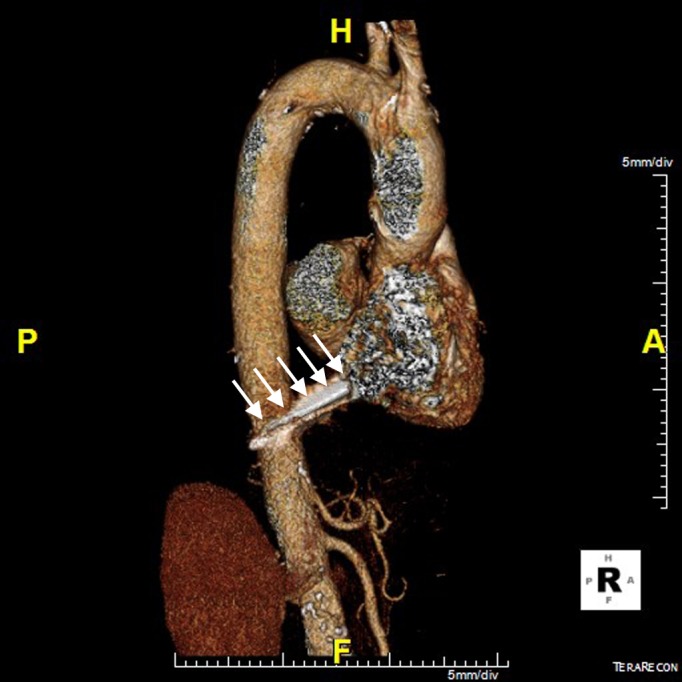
Right lateral 3D constructed contrast-enhanced CT Right lateral 3D constructed contrast-enhanced CT image showing presence of the nail puncturing the heart with the tip lying adjacent to the abdominal aorta (arrows), without evidence of rupture.
